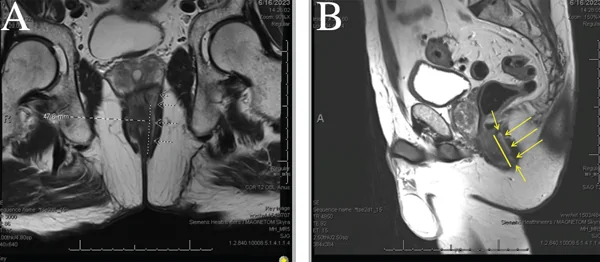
MRI scans showing positions of prostate and perianal fistula. (A) Coronal MRI image demonstrating perianal fistula (marked by arrows) of 47.6 mm in length. (B) Sagittal MRI image showing perianal fistula, marked by yellow arrows.
